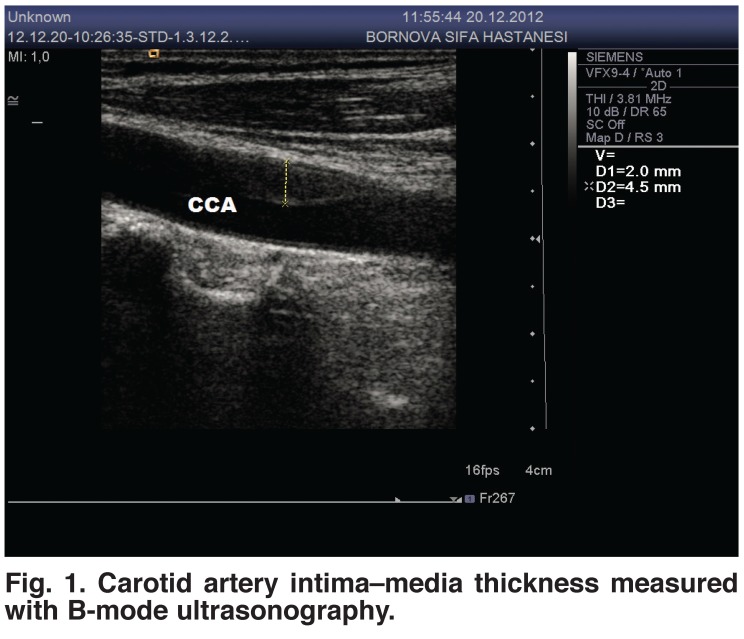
Carotid artery intima–media thickness measured with B-mode ultrasonography.
